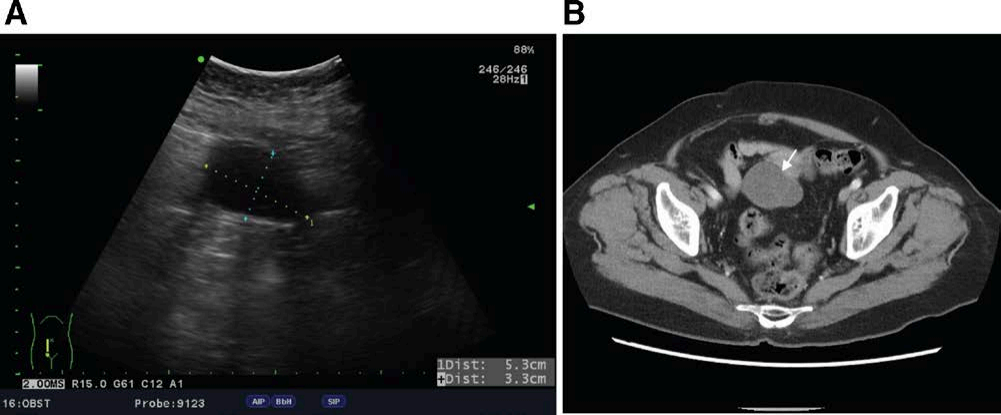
Tumor imaging. (A) Pelvic ultrasound showed a hypoechoic tumor 5.3 × 3.3 cm in size. (B) Computed tomography showed a tumor located in the intestinal region (arrow).
